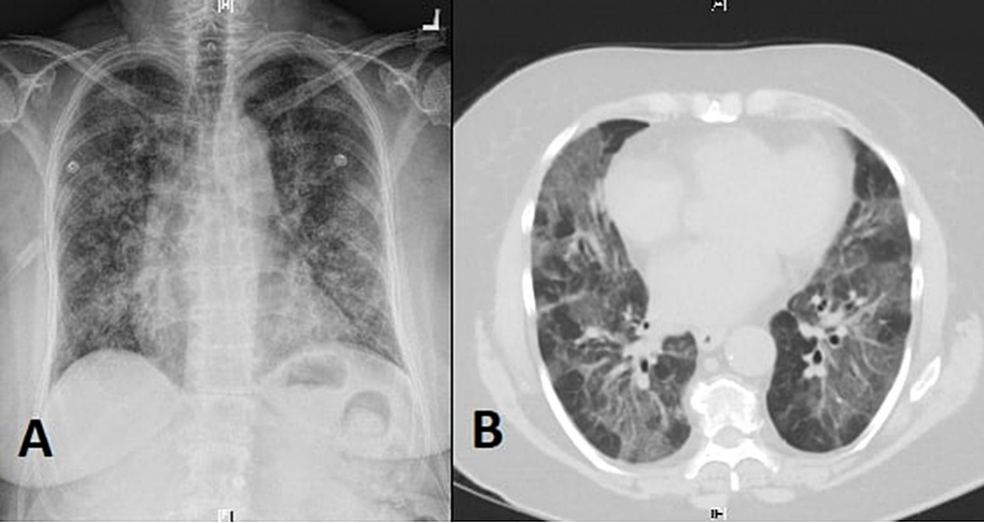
Chest X-ray showing diffuse micronodular pattern with patchy foci (A). CT scan of the chest showing bilateral extensive patchy ground-glass opacity (B).
